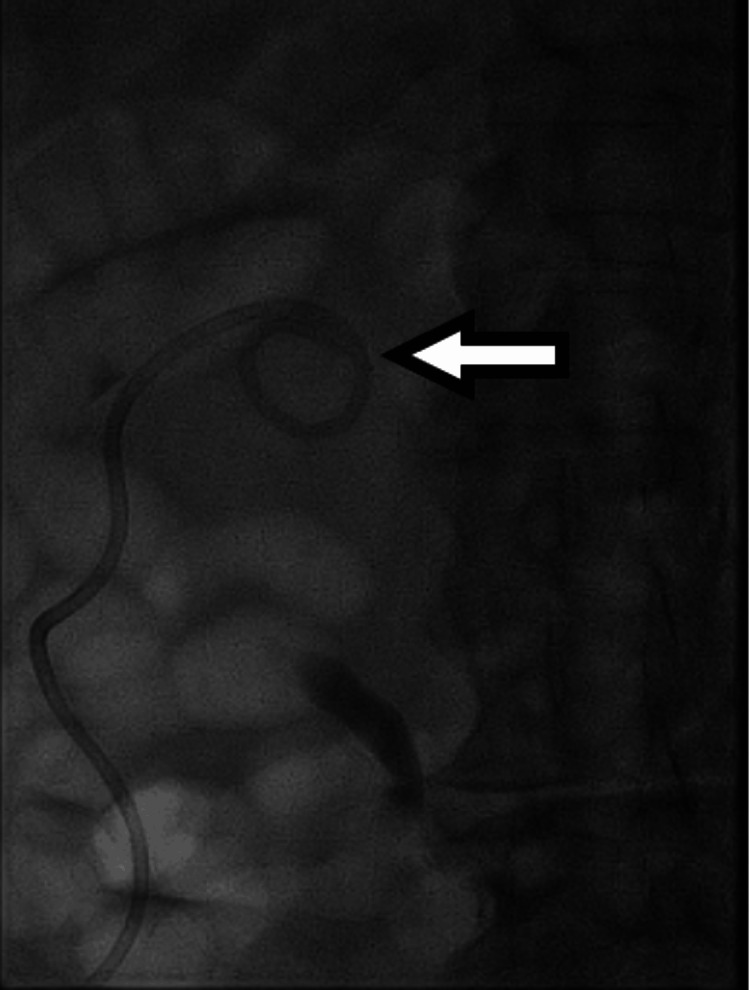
Ultrasound imaging demonstrated successful image-guided percutaneous placement of a left nephrostomy tube for moderate left hydronephrosis
